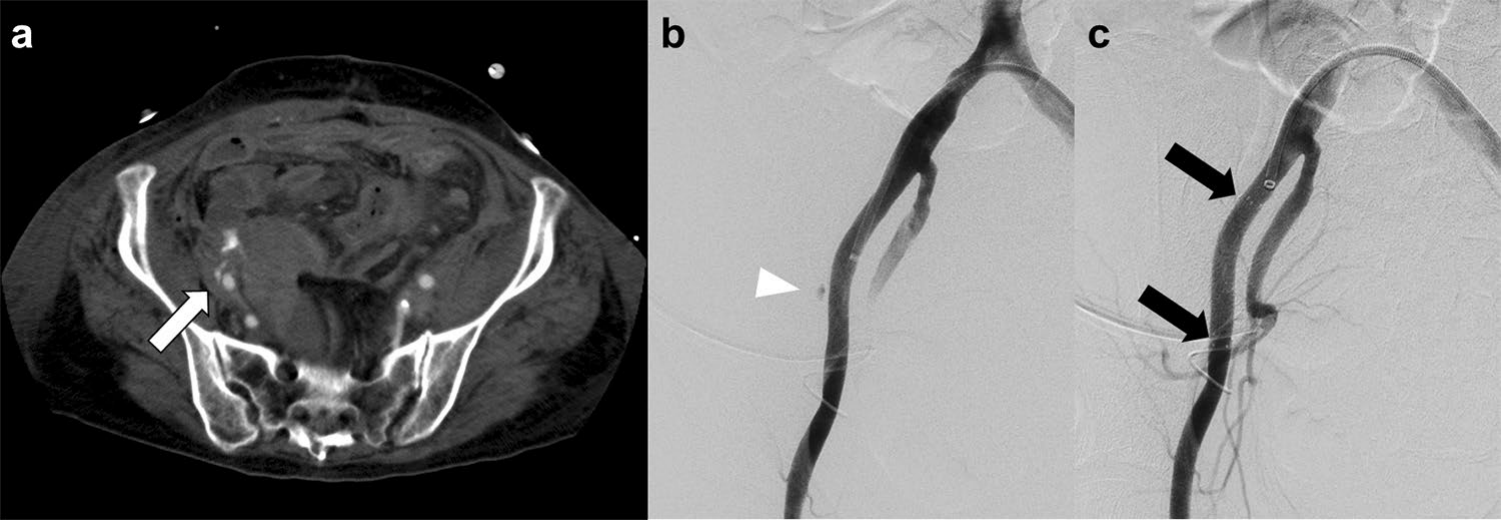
Endovascular treatment with stent graft placement for active bleeding from the external iliac artery. a CT scan shows active bleeding from the right external iliac artery (white arrow). b Digital subtraction angiography of the right external iliac artery shows active bleeding (arrowhead). c Digital subtraction angiography of the right external iliac artery obtained after angioplasty with stent graft placement (VIABAHN®, W. L. Gore & Associates, Flagstaff, Ariz) shows cessation of active bleeding. Black arrows indicate the markers of the proximal and distal edges of the stent graft. *CT* computed tomography
